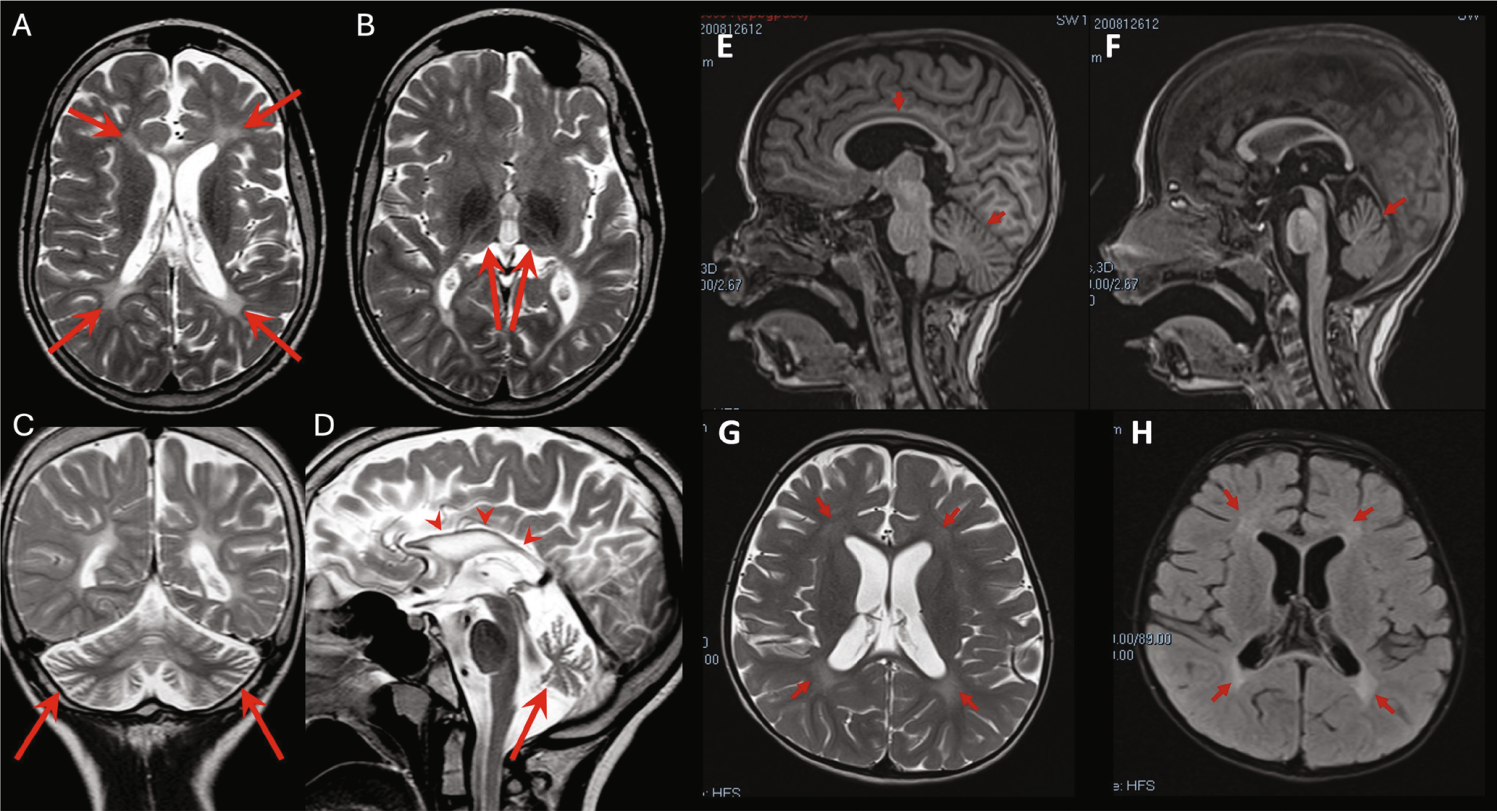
In the left panel, brain MRI scan of affected proband of Family 1; axial (A, B), coronal (C), and sagittal (D) T2-weighted sequences showing volumetric reduction and hyperintensity of periventricular and deep white matter (arrows in A). The thalami are atrophic and show contextual laminar hyperintensity (arrows in B). Hemispheric and vermian cerebellar atrophy is also present (arrows in C and D), as well as a markedly thinned corpus callosum (arrowheads in D). In the right panel, brain MRI scan of affected proband of Family 2; T1-weighted sagittal image (E, F), T2-weighted axial image (G), and FLAIR axial image (H) show mild dilatation of the lateral ventricles with diffuse hypomyelination, more pronounced in the frontal and occipital periventricular white matter (arrows in G and H), thinning of the corpus callosum (arrow in E), and mild cerebellar atrophy (arrows in E and F).
